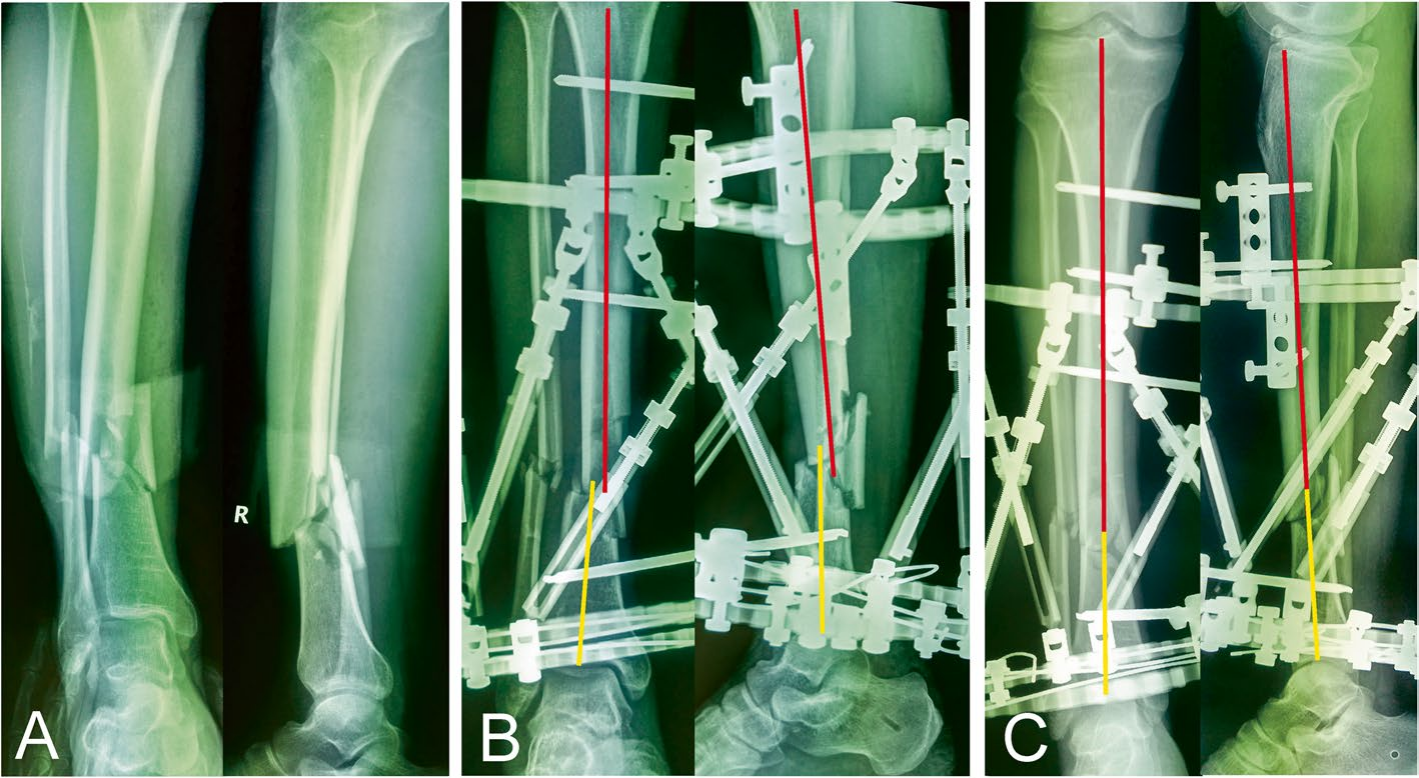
A 52-year-old man with traumatic multidimensional deformity in tibia treated by the hexapod external fixator, performing staged correction trajectory, this patient had one repeated X-ray exposure after the first postoperative X-ray and a three-day of deformity correction process. a Traumatic X-rays. b X-rays after surgery. c X-rays after the first correction
